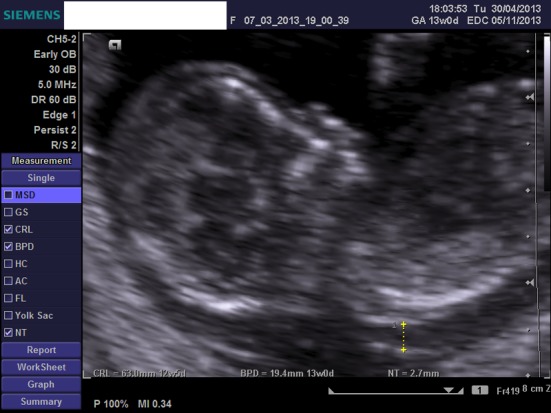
Ultrasound image at 12 weeks, showing the ductus venosus Doppler.
